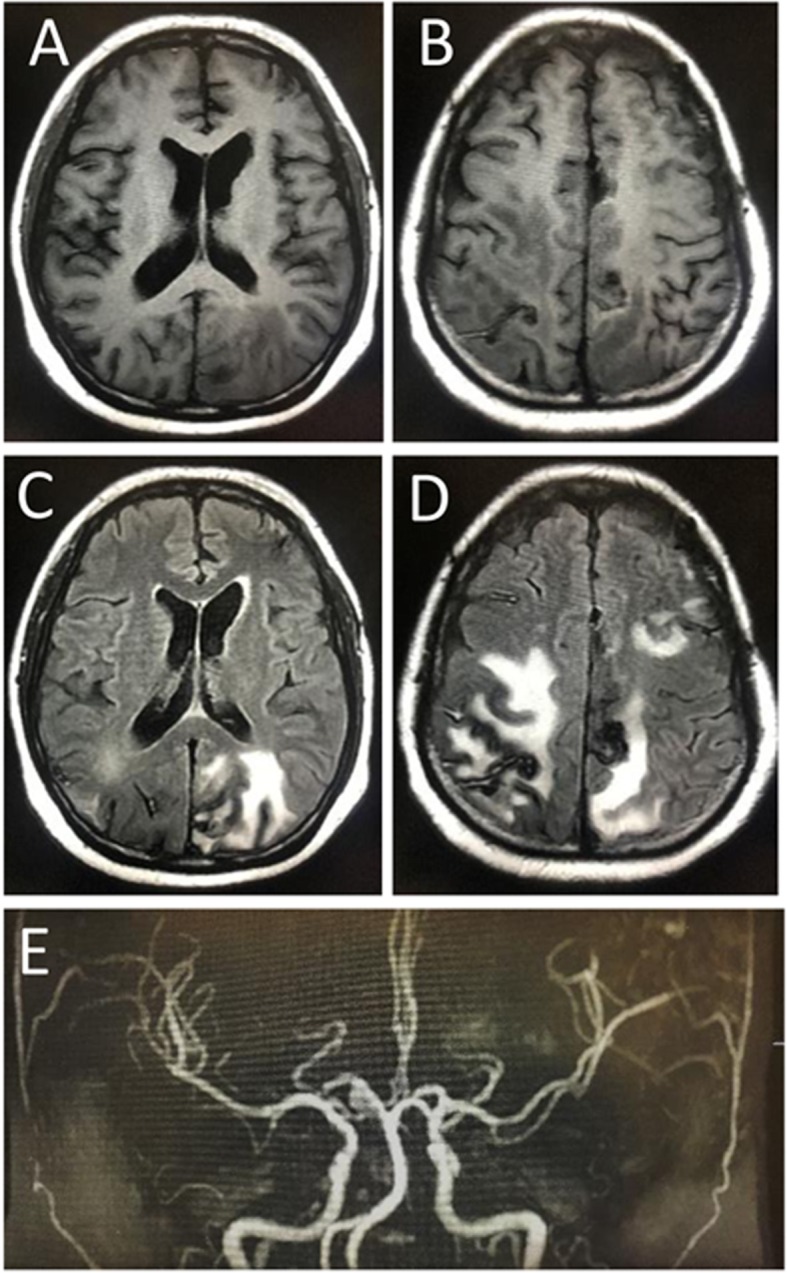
MRI brain images from follow-up examination. a-b The axial T1-weighted images. c-d The axial T2-weighted flair images show multiple nodules or mass shadow. e The MRA image for aneurysms
